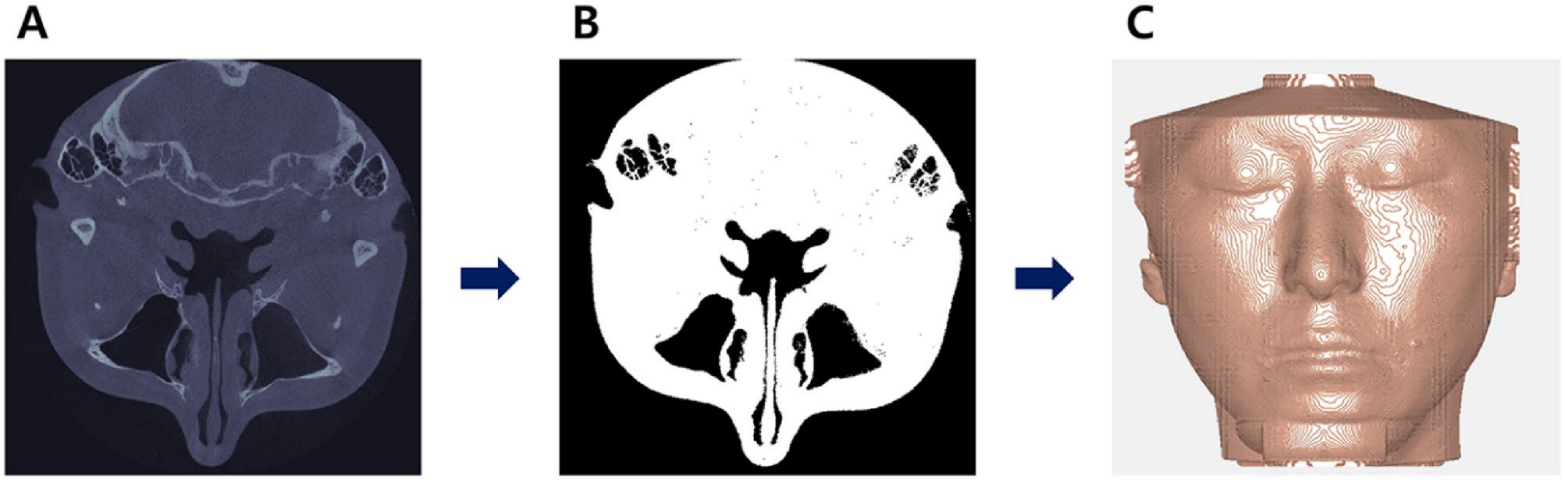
Image processing sequence. (A) Axial view of cone-beam computed tomography image. Hard tissues appear white, and soft tissues appear black or gray. (B) Automatic binarization using the Otsu method. Soft tissue images appear white. (C) Surface reconstruction using the marching cube algorithm. Contour lines are formed.
